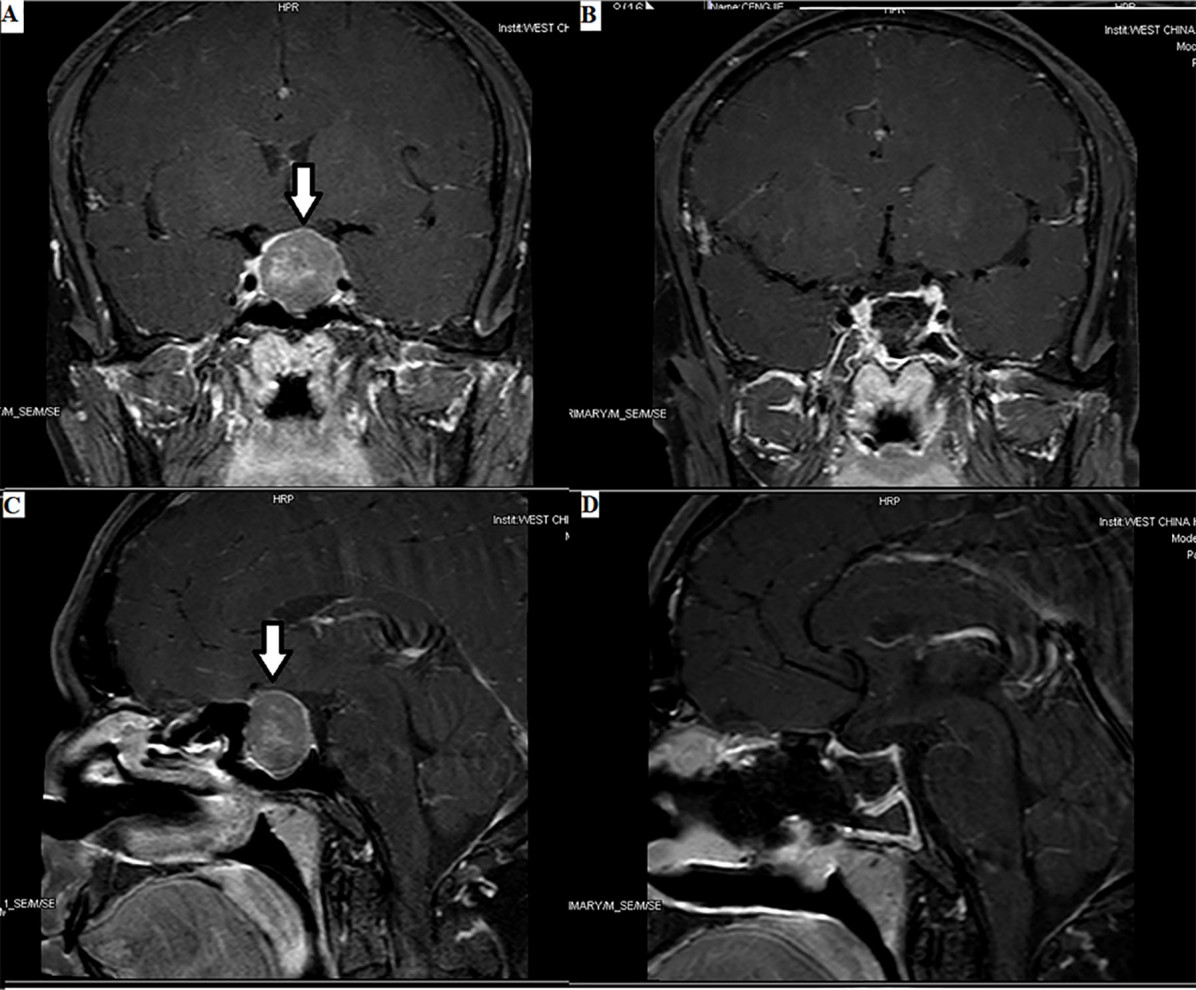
Enormous TSH PitNET: 1. Preoperative magnetic resonance imaging (MRI) (A, C): solid tumor occupying the saddle area, with a size of 1.8×2.4×2.5 cm, as indicated by the arrows. 2. Postoperative MRI (B, D): low signal shadow in the sella region.
